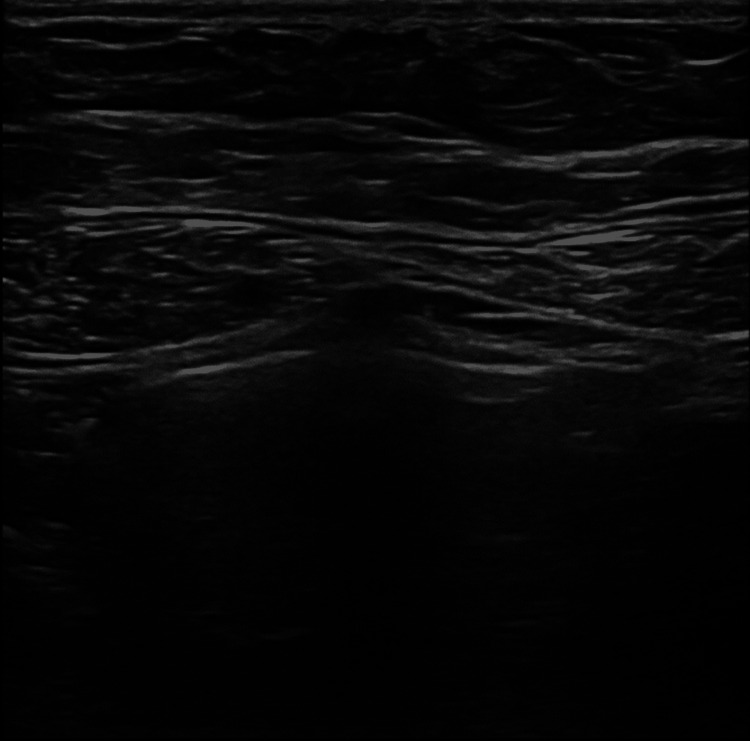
Complete resolution of the hematoma after 12 weeks, with normal findings on ultrasound.
